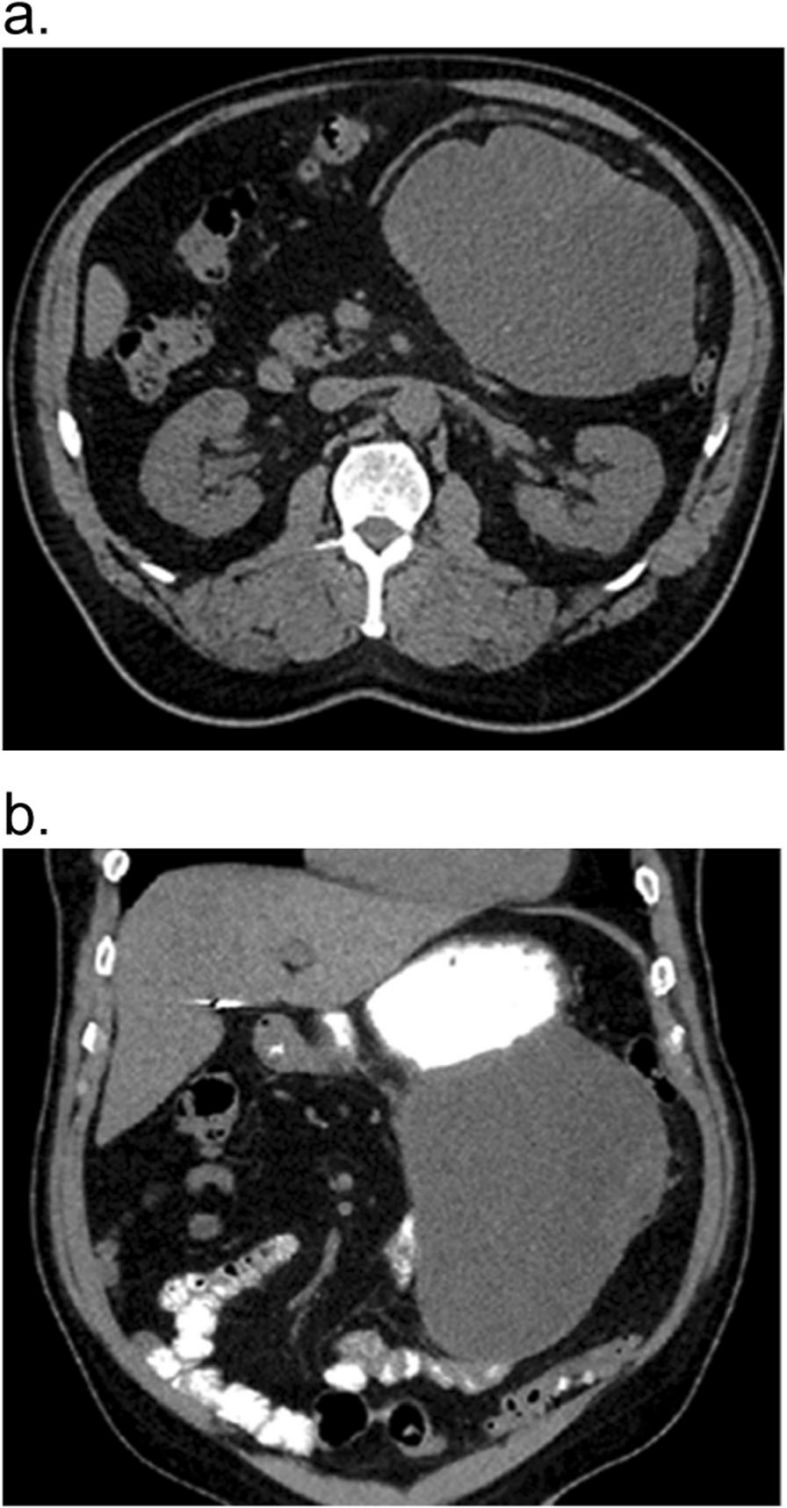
CT-scan of Gastrointestinal Stromal Tumor (GIST) Prior to Surgery: a axial, b coronal
